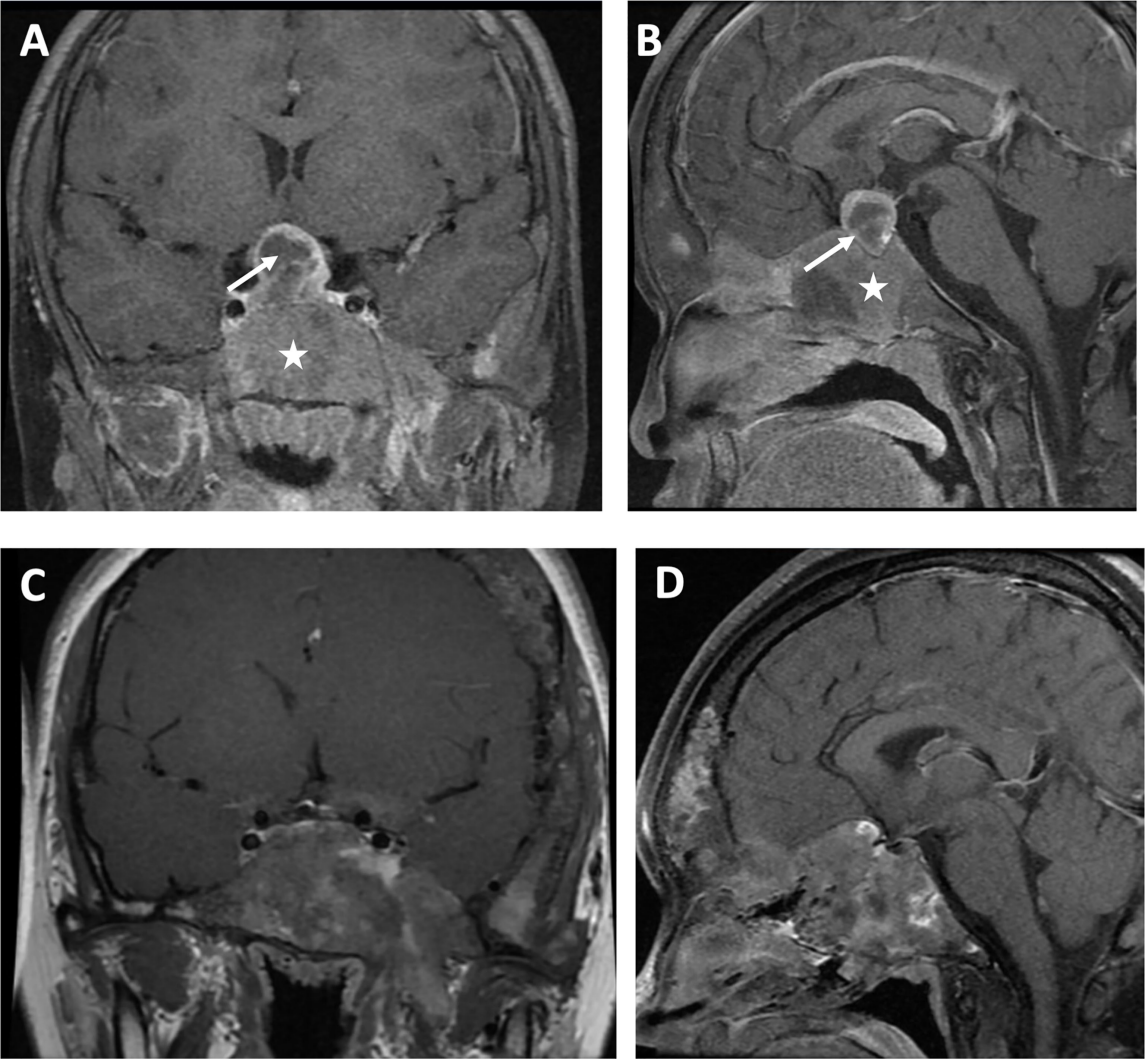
Visualization by MRI in the coronal view (A) and sagittal view (B) of a macroadenoma (arrow) surrounded by cranial fibrous dysplasia (star) in a patient with MAS and GH excess before surgery and pharmacologic treatment. Coronal view (C) and sagittal view (D) after surgery and pharmacologic treatment.
